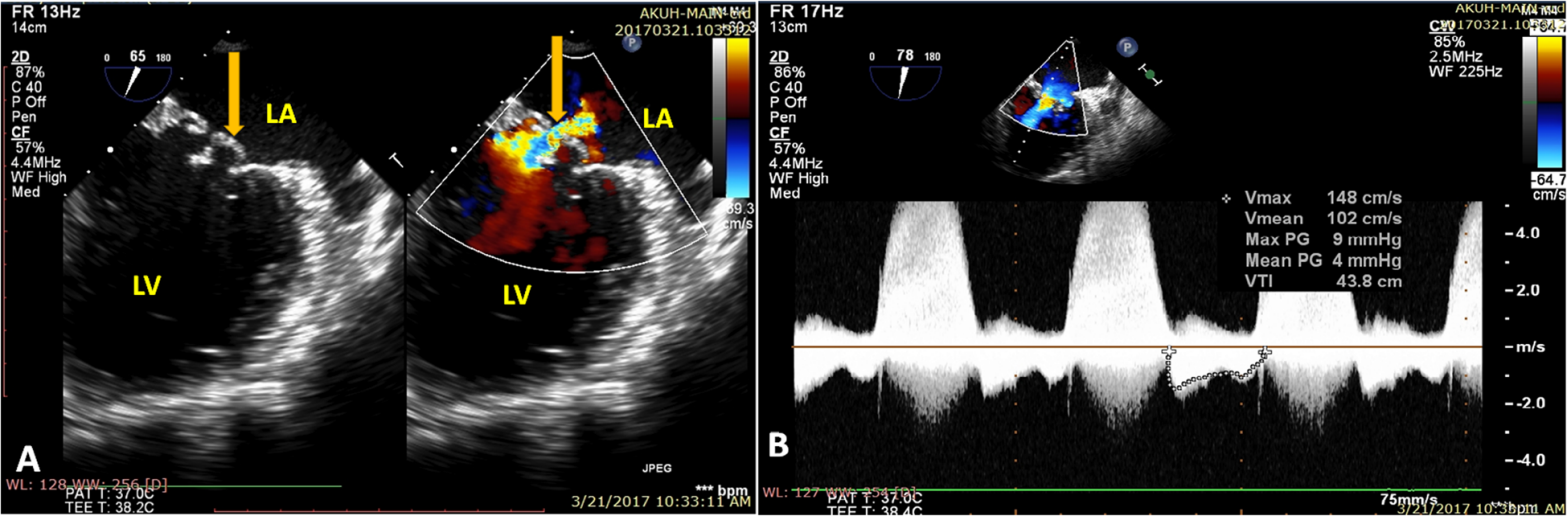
a The color Doppler flow showing that the aneurysm was filled with flow during systole (yellow arrow) and the color flow in the cystic mass was communicated with the left atrium. b Continuous wave Doppler revealing the aneurysm causing hemodynamic disturbance and an increase of mean pressure gradient. LA: Left Atrium, LV: Left Ventricle
